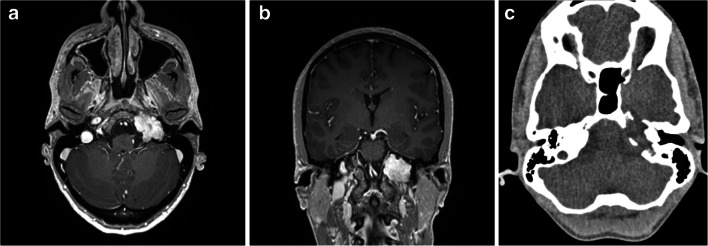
Preoperative T1 Gd+ MRI demonstrating the chondrosarcoma in the petrous apex left side on axial a and coronal b cuts. Preoperative CT demonstrating the chondrosarcoma in the petrous apex left side on axial c cuts
